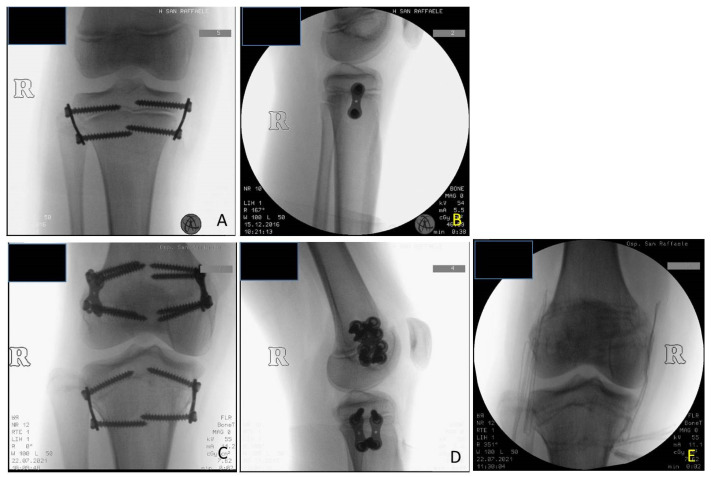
BWS (Beckwith-Wiedemann syndrome) female patient with LLD (leg length discrepancy) right: (A) at age of 9.8 years PTE (temporary proximal tibia epiphysiodesis) was performed using 8-plates; X-ray in anteroposterior projection (B) X-ray in lateral projection; (C) at age of 10.7 years DFE (distal femur epiphysiodesis) was performed using quad-plates, X-ray in antero-posterior projection; (D) X-ray in lateral projection; (E) at age of 14.3 years removal of hardware by absent LLD, X-ray in antero-posterior projection showing closed physis. (8-plate^R^ and quad-plate^R^; Eight Plate Guided Growth System+, Orthofix Medical Inc.© Lewisvill, TX (USA)).
